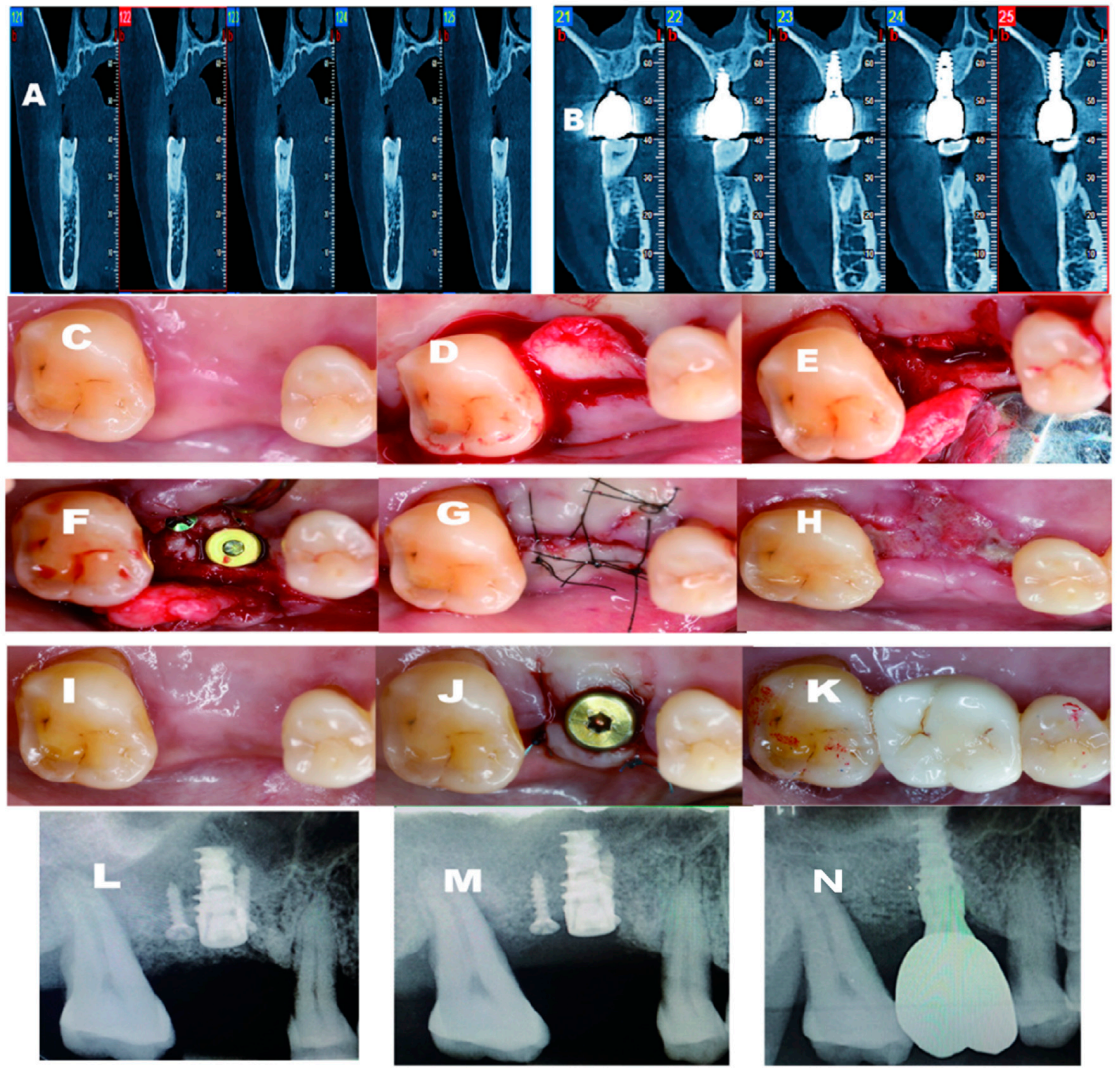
(A) Pre-operative CBCT indicates that large palatal defect; (B) 3 years follow-up CBCT. shows excellent bone regeneration; (C) Pre-operative-occlusal view; (D) Palatal flap elevated; (E) Ridge split initiaed; (F) Fixture inserted; (G) After sutured; (H) 2 weeks post-op; (I) 4-month post-opertative; (J) Second stage surgery and healing abutment installed; (K) Final crown delivered; (L) Periapical X-ray post-operative immediately; (M) Periapical X-ray 4 months after the surgery; (N) Periapical X-ray after crown delivery.
